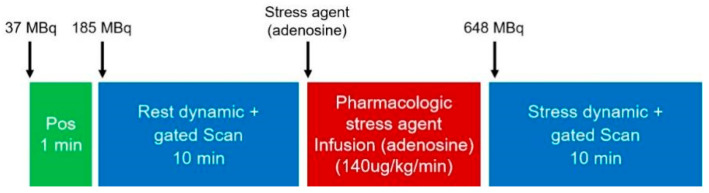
CZT-SPECT CFR continuous rapid imaging protocol.
